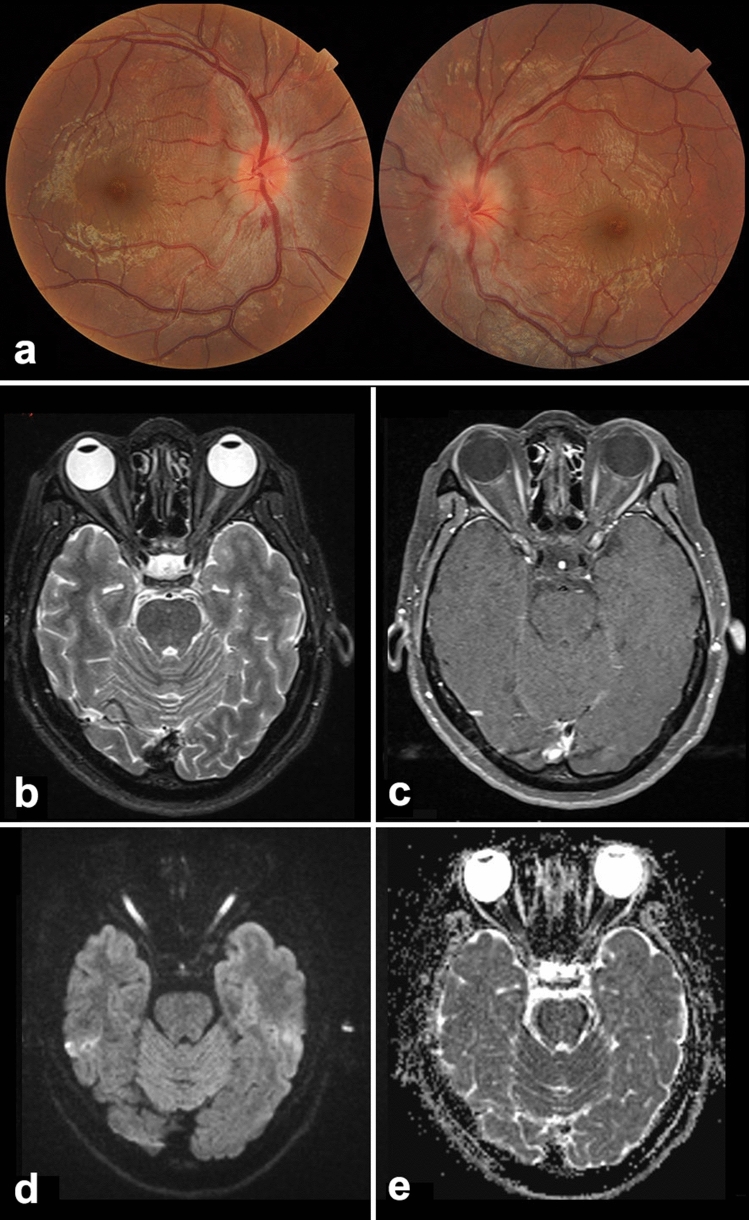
A 20-year-old young man with acute profound bilateral visual loss, seronegative for myelin oligodendrocyte glycoprotein and aquaporin 4 antibodies. (a) The disc is swollen bilaterally with hemorrhage; (b–e) Orbit magnetic resonance imaging (MRI) at the acute stage showing mild hyperintensity of the optic nerves on T2-weighted imaging (WI) (b), no enhancement on T1WI after contrast (c); diffusion-weighted MRI (d) showing extreme hyperintensity of bilateral optic nerves with reduced apparent diffusion coefficient (e) value, indicating severe damage of the nerve. The best-corrected visual acuity at the 3-year follow up was 20/400 OU.
